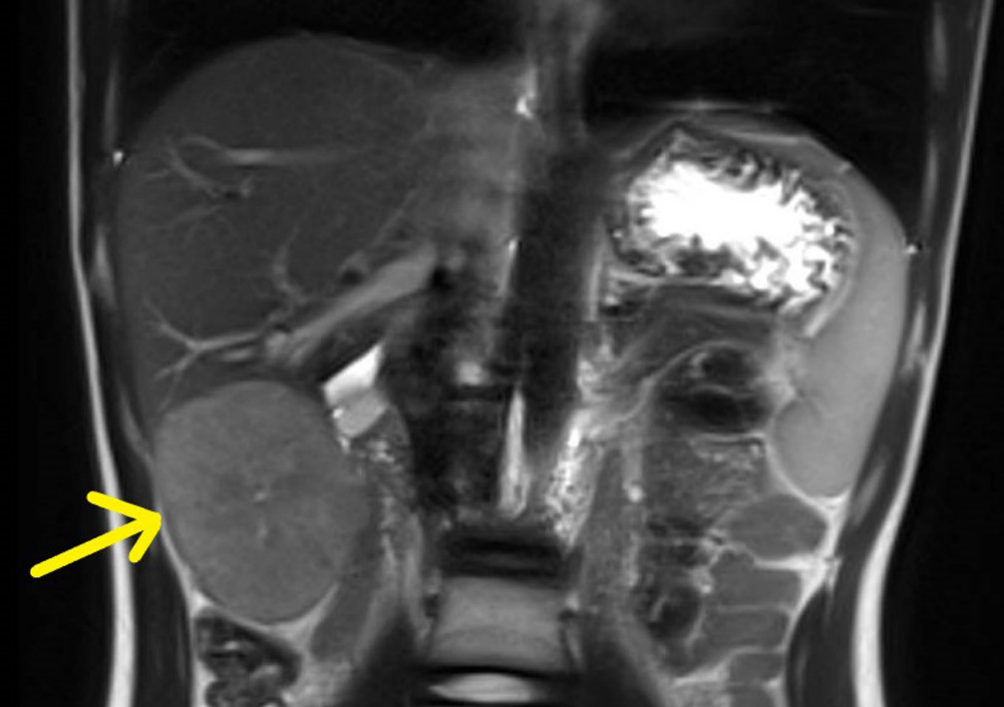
Magnetic resonance imaging of 7.5 by 6.5 by 6 cm well-defined mass in the inferior right hepatic lobe (yellow arrow). The mass does not extend to the liver hilum and does not involve the portal vein or inferior vena cava. Spleen span is normal at 11 cm.
